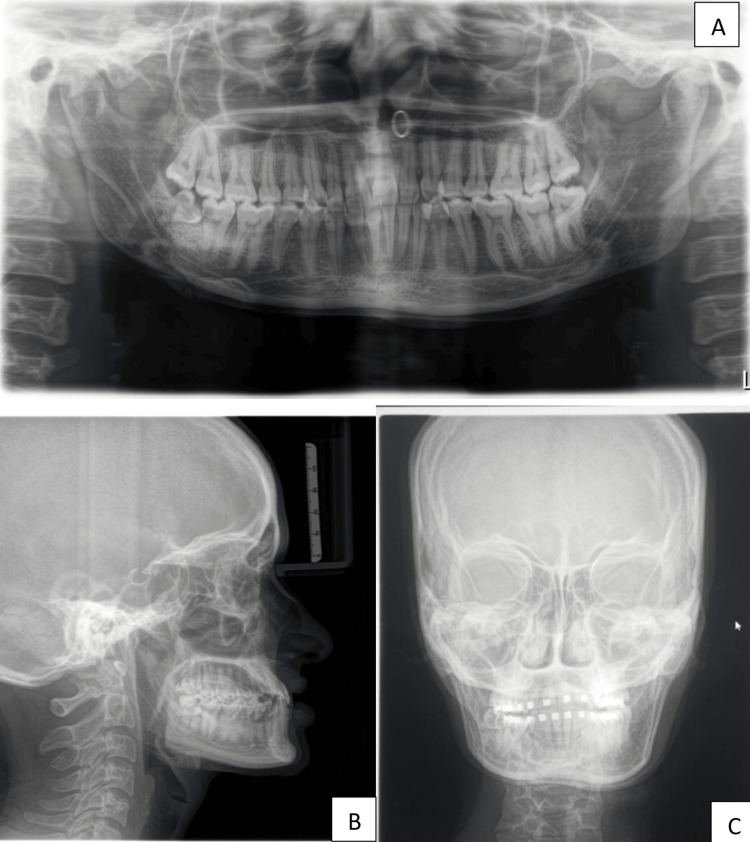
Radiographic evaluation of the patient (A) Orthopantomogram showing reduced ramus and corpus length on the right side along with an impacted third molar; (B) Lateral cephalogram demonstrating a skeletal Class III jaw relationship with a concave soft tissue profile (SNA=82°, SNB=86°, ANB=–4°); (C) Posteroanterior cephalogram confirms facial asymmetry. SNB: Sella-Nasion-Point B angle; ANB: Point A-Nasion-Point B angle.
